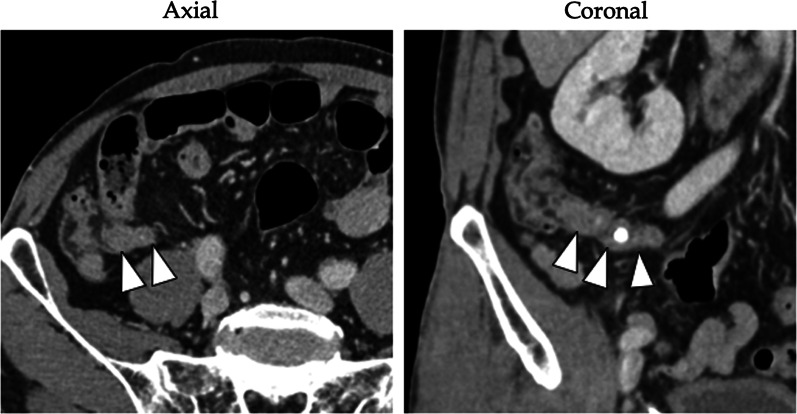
Contrast-enhanced CT scan. The CT scan revealed focal wall thickening of the appendix (indicated by arrowheads) accompanied by coprolite, but without notable enlargement of perienteric lymph nodes or apparent congenital anomalies
